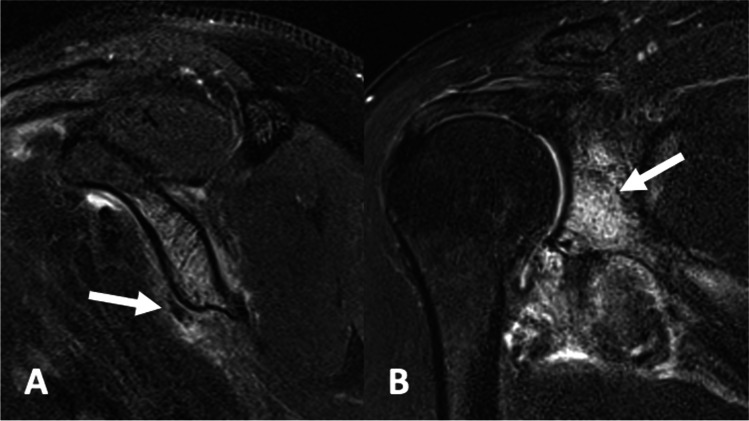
A 56-year-old female (from Fig. 1A). A Sagittal T2-weighted sequence with fat saturation shows diffuse bone marrow edema within the glenoid and a focus of capsular calcification adjacent to the antero-inferior glenoid (arrow). B Coronal proton density with fat saturation image demonstrates diffuse bone marrow edema within the glenoid (arrow) and florid capsular/pericapsular edema
